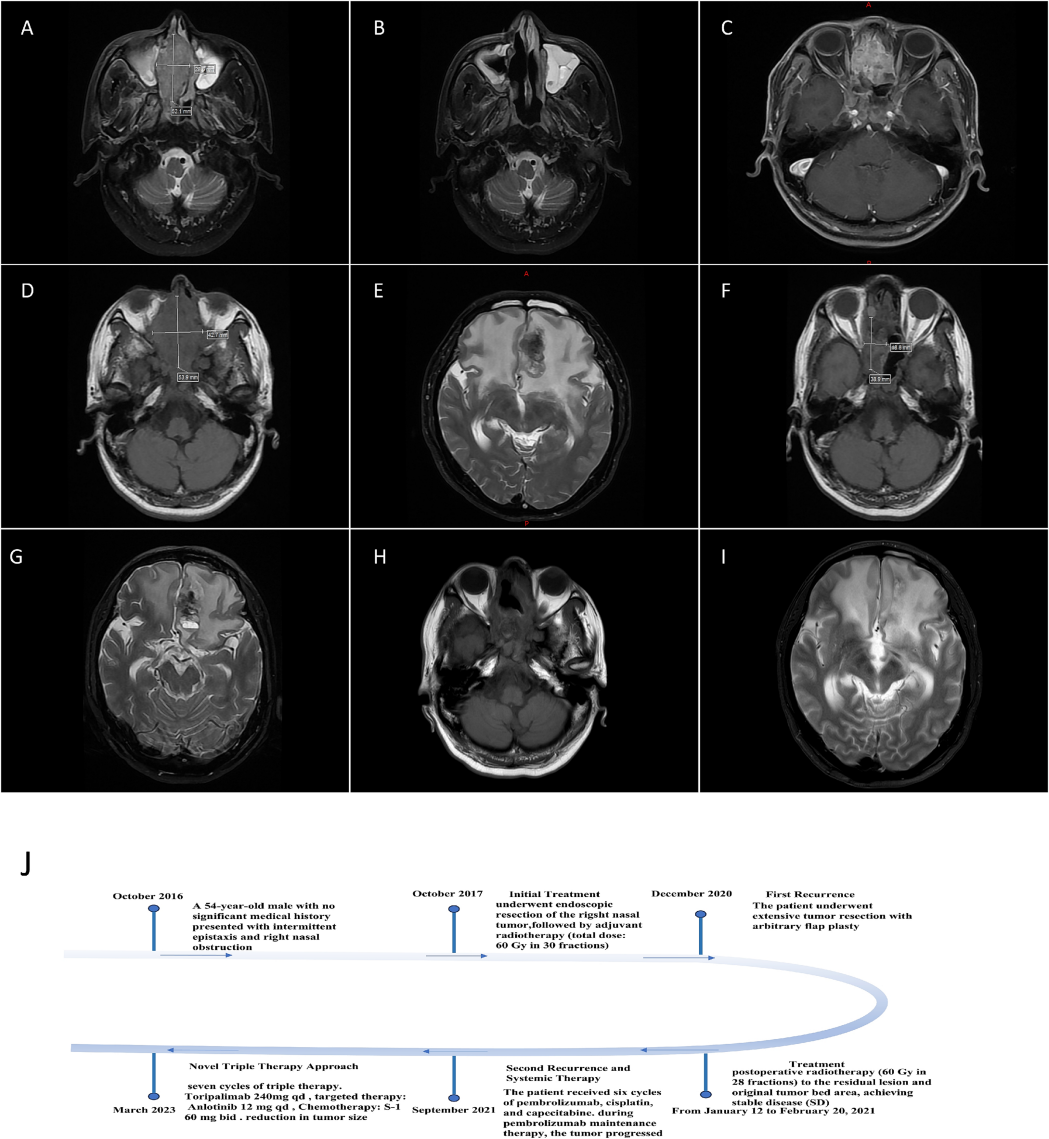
MRI Imaging of ITAC. (A) Preoperative MRI: this image demonstrates a moderately enhancing mass within the right nasal cavity with poorly defined margins. The lesion extends posteriorly to involve the nasopharyngeal cavity, causing narrowing. There is a leftward deviation of the nasal septum, and the signal intensity within the lesion is heterogeneous. (B) Postoperative MRI: the image shows the absence of the right middle and superior nasal conchae, as well as a partial resection of the nasal septum. Most of the irregular soft tissue mass previously occupying the right nasal cavity has been surgically removed, with residual post-surgical changes visible. (C) MRI after surgery and radiotherapy: the lesions in the ethmoidal sinus at the upper margin of the operative area had increased in size compared to before, with invasion of the frontal lobe base and the anterior part of the brain. Tumor recurrence with a high likelihood of invasion into adjacent structures was considered. (D) The mass in the nasal cavity surgical area has increased in size compared to the previous examination, with an expanded range of intracranial invasion. The extent of invasion has also increased in the medial walls of both orbits, the orbital apices, and both optic nerves. (E) The extent of perilesional brain edema has increased. (F) Follow-up after comprehensive treatment for recurrent nasopharyngeal malignancy: The previous findings of middle turbinate defect and partial absence of the nasal septum remain unchanged. The soft tissue mass in the surgical area has decreased in size, with an ill-defined border. The invasion of the frontal lobe and basal meninges at the upper margin of the lesion has shown some improvement. There is also improvement in the invasion of the clivus, medial walls of both orbits, orbital apices, optic nerves, pterygoid processes, and the internal and external pterygoid muscles on both sides. (G) Reexamination MRI: the scope of cerebral edema was reduced. (H) MRI review in August 2023 indicated a slight enlargement of the soft tissue mass in the operative area. (I) The degree of bilateral cerebral edema has worsened compared to the previous imaging examination. (J) Patient Treatment Schedule.
